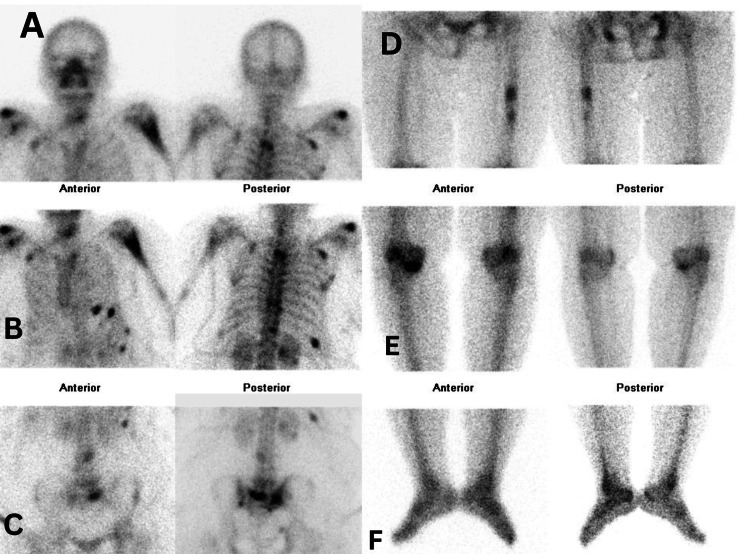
Bone scan showing multiple skeletal metastasis The image shows a body scintigraphy scan demonstrating extensive skeletal metastases. Anterior and posterior views reveal areas of abnormal uptake. (A) skull and shoulder girdle: physiologic uptake without any evidence of focal metastatic lesions; (B) Thoracic spine and ribs: Multiple areas of radiotracer accumulation in the thoracic vertebrae and ribs, consistent with metastatic disease; (C) Lumbar spine and pelvis: Marked uptake in lumbar vertebrae and sacroiliac joints, suggestive of tumor infiltration; (D)Pelvic girdle and femur: Asymmetric distribution in these areas indicates metastatic spread; (E)femoral shaft: Bilateral focal uptake in femoral shafts raises concern for cortical involvement or marrow involvement' (F)Distal lower extremities: physiologic distribution of tracer in distal tibia and feet with no abnormal foci.
